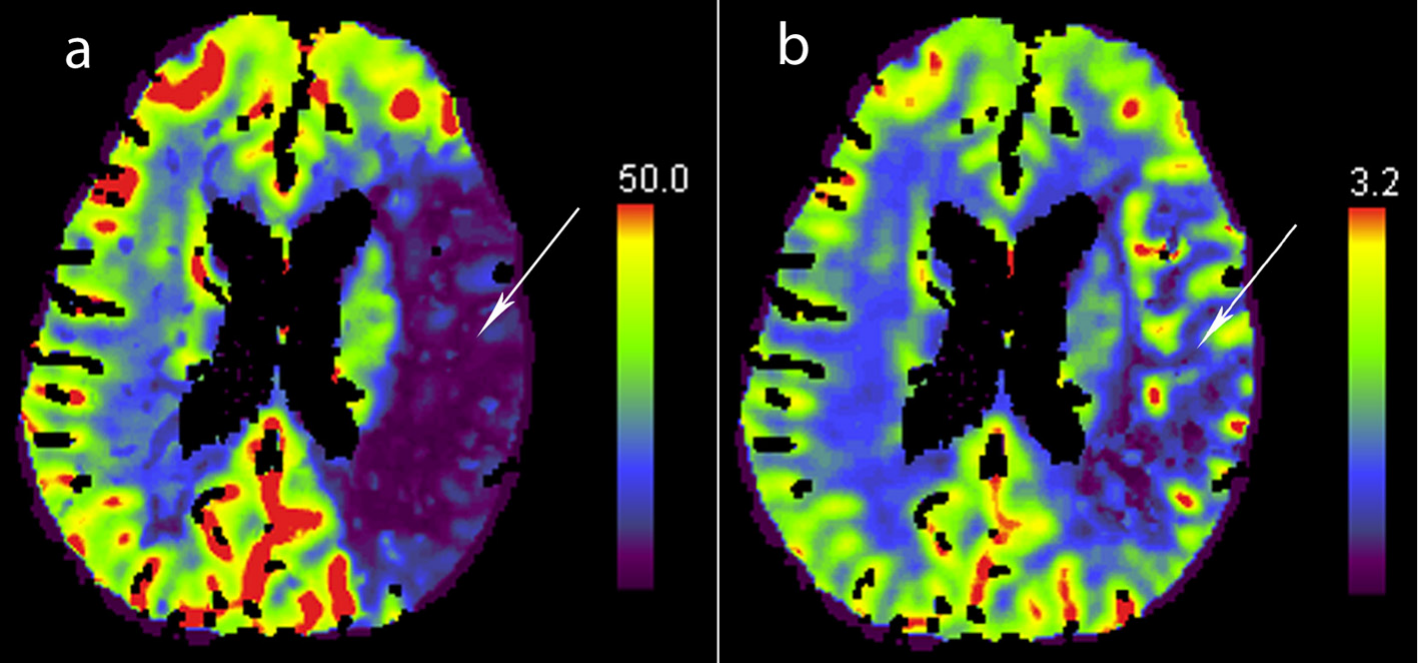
CT perfusion imaging demonstrating area of diminished CBF (a; arrow) with relatively normal CBV (b; arrow) suggesting evidence of salvageable penumbra.
